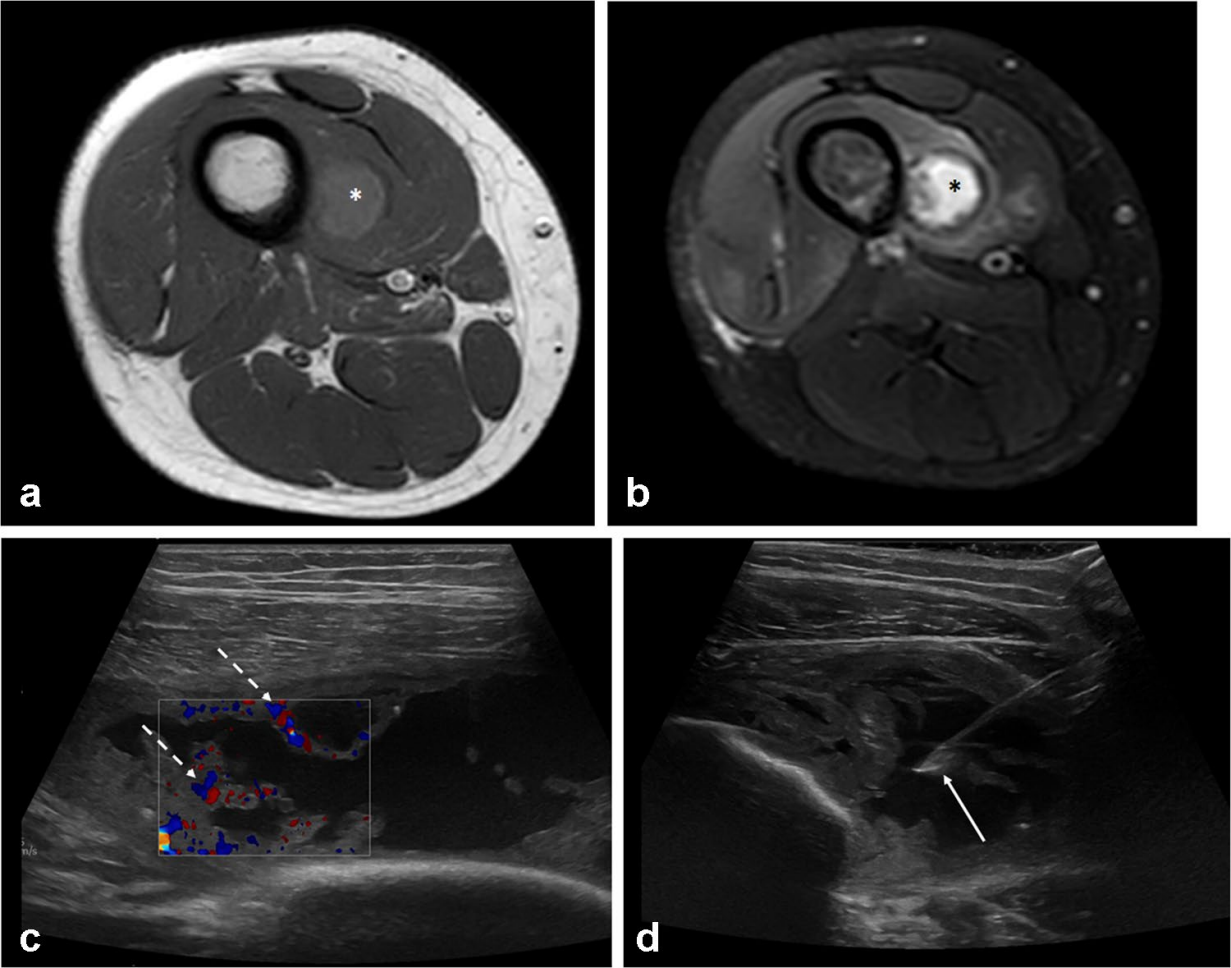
Twelve-year-old boy with right thigh pain and swelling. T1 (a) and T2 FS (b) MRI images revealed features of pyomyositis in the abscess phase, within the vastus intermedius muscle (asterisks). A mild hyperintense signal intensity on T1 sequence can be noted, possibly related to the proteinaceous content of the infected fluid-collection. Marked hyperemia of the abscess walls can be detected on color-Doppler ultrasound (c, dotted arrows). The patient underwent ultrasound-guided aspiration (d) with an 18G needle (arrow), for microbiological assessment. The diagnosis of methicillin-resistant *Staphylococcus aureus* infection was obtained
